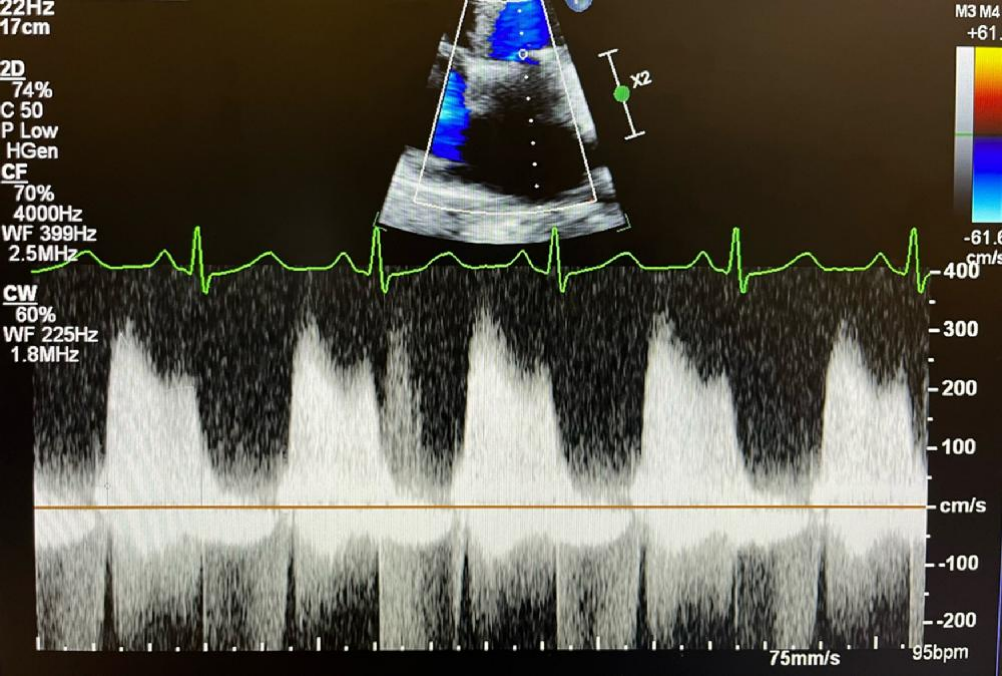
Pre-operative transthoracic echocardiography imaging, continuous wave Doppler parallel to the mitral inflow in apical four-chamber view. Peak and mean mitral valve gradients are measured as 34 and 19 mmHg at a heart rate of 95 b.p.m., respectively.
